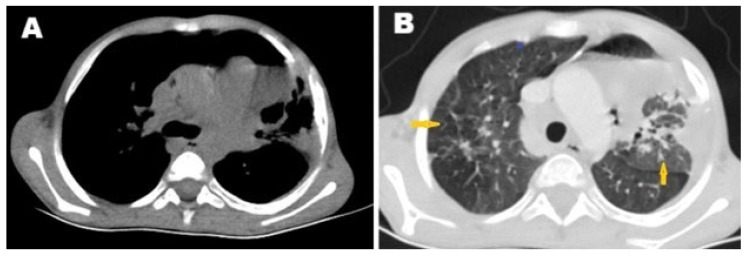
Selected axial images of computed tomography of (A) chest window, showing left lung lesion and (B) lung window, showing left lung consolidation with numerous centrilobular nodules with opacified distal bronchioles forming the tree-in-bud sign (arrows) with minimal left pleural effusion.
